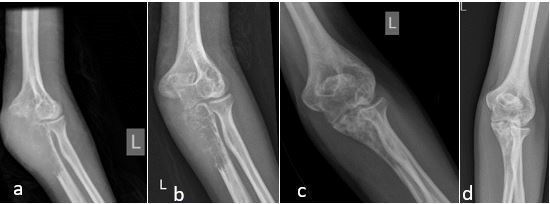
Left elbow radiographs showing (a) lytic lesion with soft tissue component in the proximal ulna with progressive bone formation in (b) after 1 cycle of chemotherapy (c) after 2 cycles of chemotherapy and (d) post radiotherapy and 4 cycles of chemotherapy.
